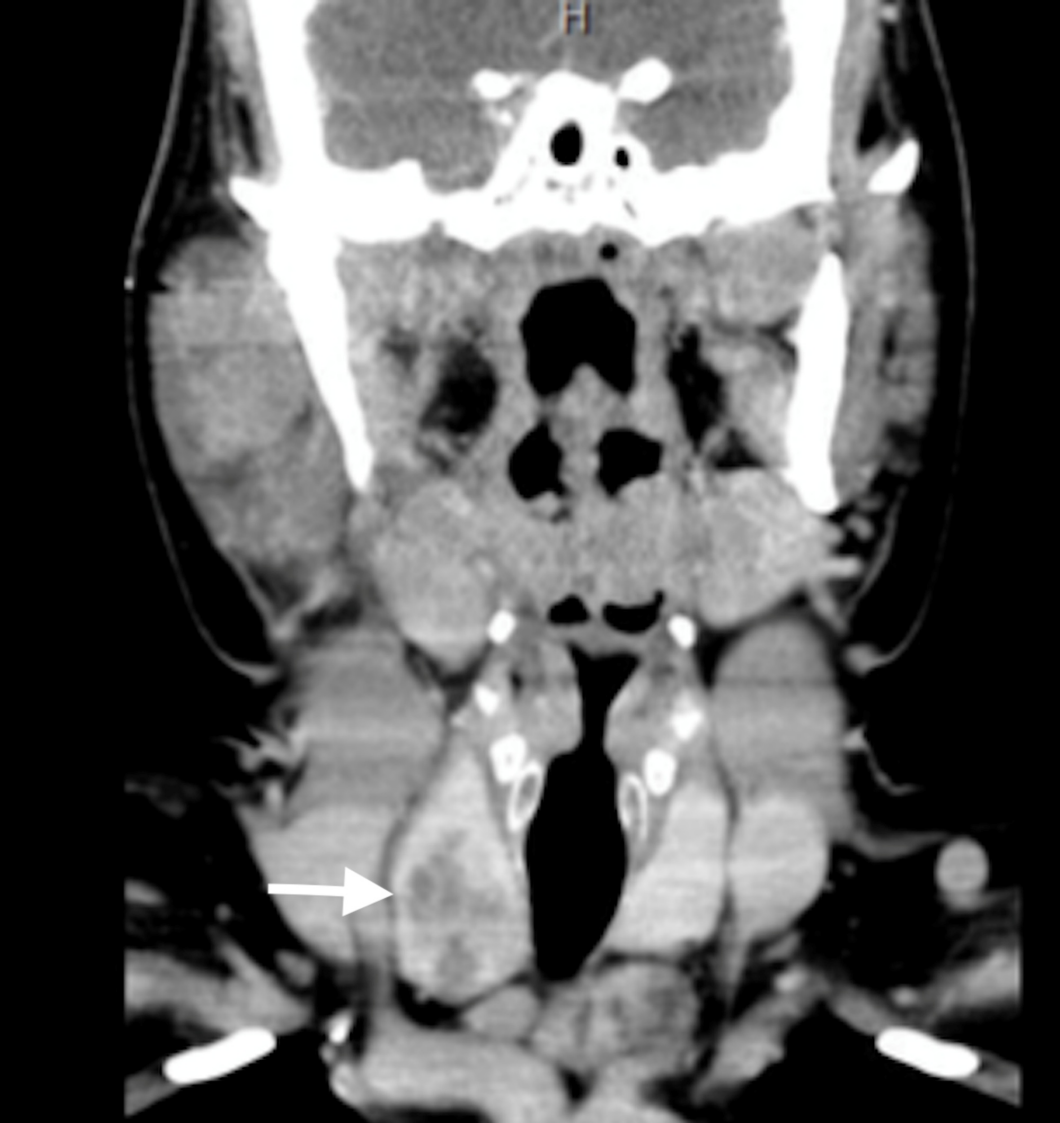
Contrasted CT neck image in the coronal plane The image shows incidental calcifications in the right thyroid lobe (arrow) CT: computed tomography
